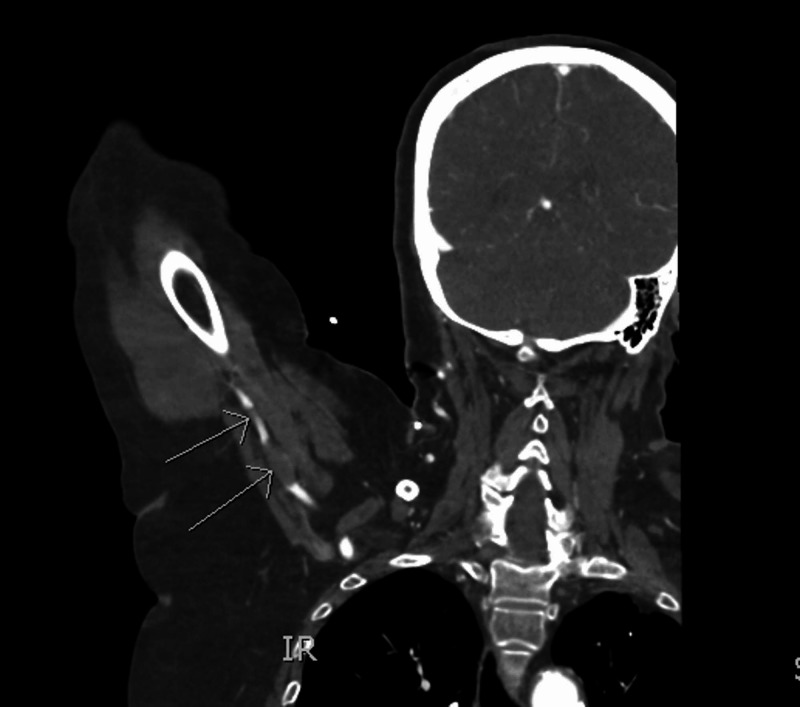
Coronal computed tomographyCT angiography of the right axillary artery demonstrating lack of contrast flow and thromboembolism (arrows)
